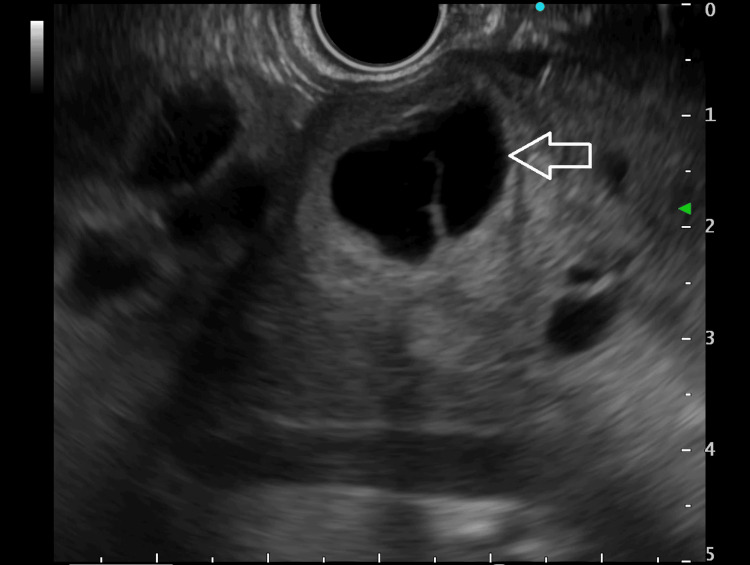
Endoscopic ultrasound showing multiple hypoechoic lesions (arrow) in the left liver lobe with thin septae and one of them abutting the gastric wall
